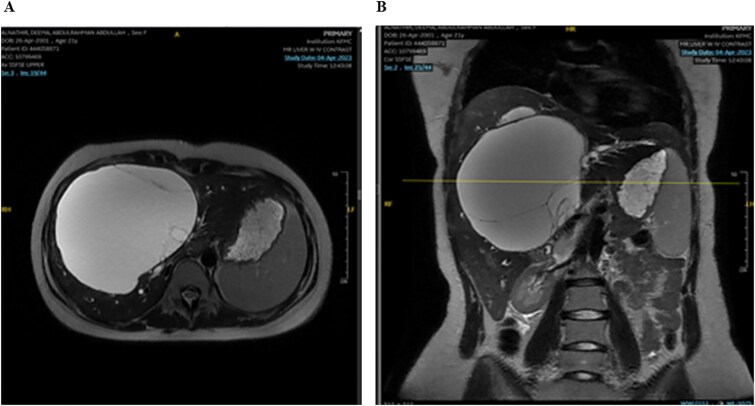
MRI of the liver: Demonstrating the mass in T2. Mild splenomegaly was noted without focal lesions. A) Transverse view, B) longitudinal view.
